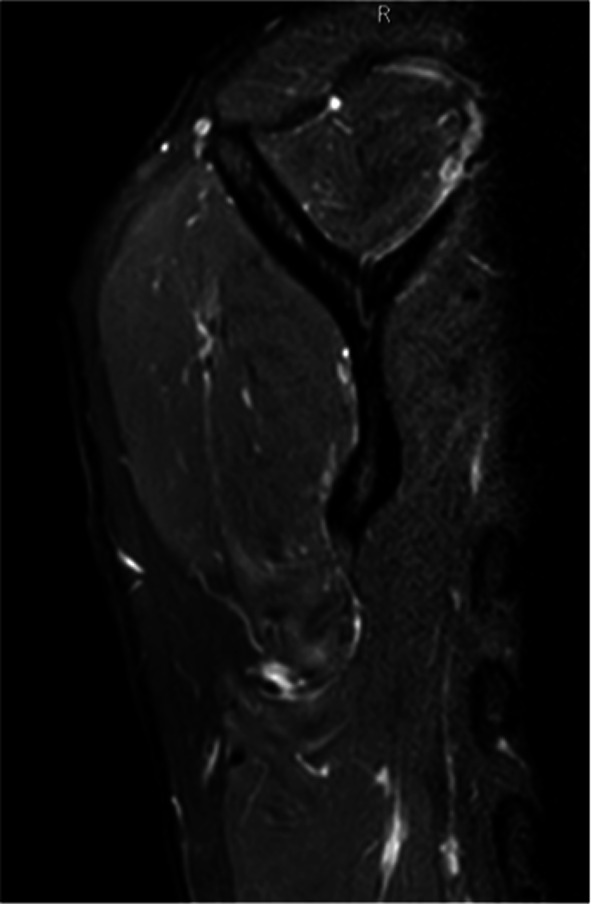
Edematous and atrophic right teres minor on magnetic resonance imaging of shoulder
